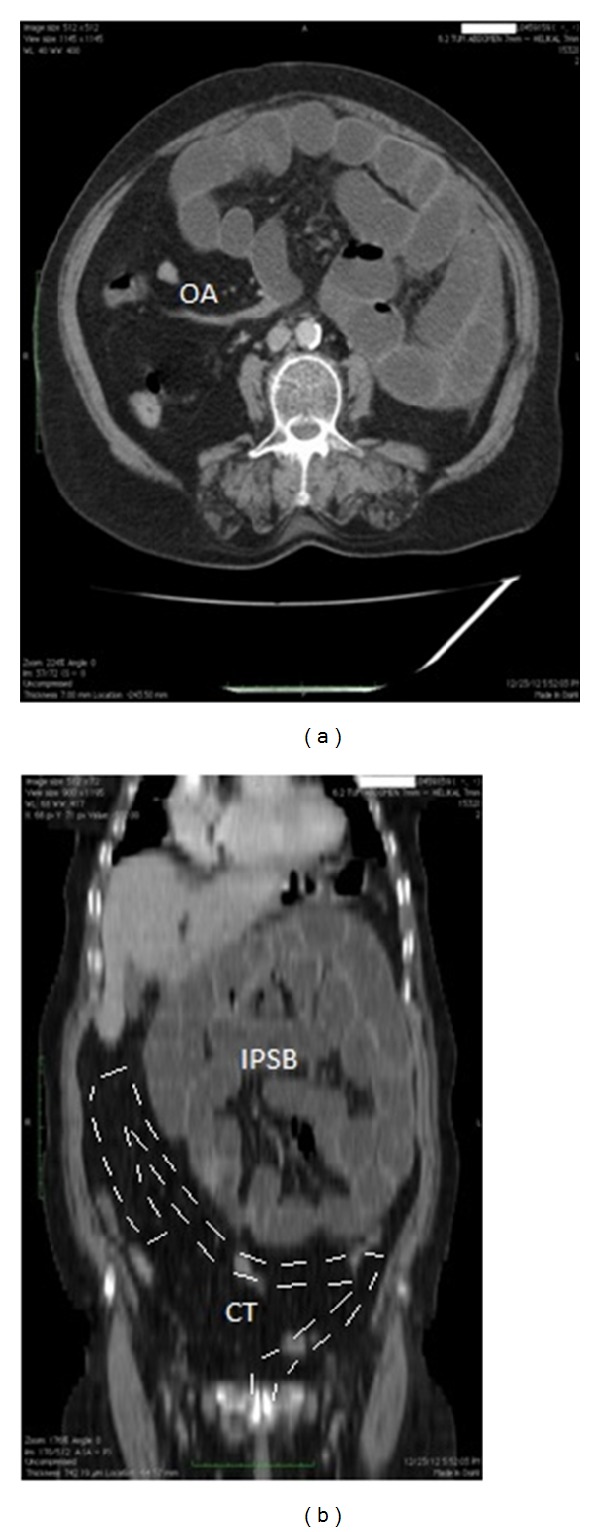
CT images demonstrating intestinal malrotation. On axial image (a), obstructed area (OA) indicates the obstructed part of terminal ileum. On coronal (b) image intraperitoneal small bowel (IPSB) is indicated in upper abdomen. Colon trace (CT) is oriented with (-) symbol.
